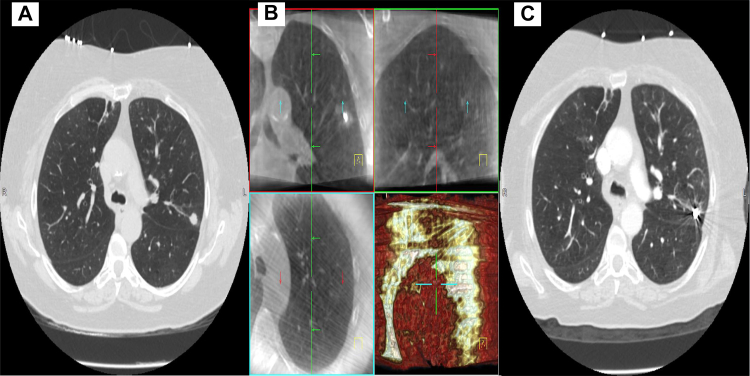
A-C, Representative imaging showing stability of indocyanine green-soaked fiducial marker (ICG-F) after placement during shape-sensing robotic-assisted bronchoscopy (ssRAB). A, Chest CT scan showing a left upper lobe peripheral pulmonary lesion. B, Cross-sectional imaging after ICG-F placement near the PPL via ssRAB. C, Stability of ICG-F after repeat chest CT 6 mo after ICG-F placement.
